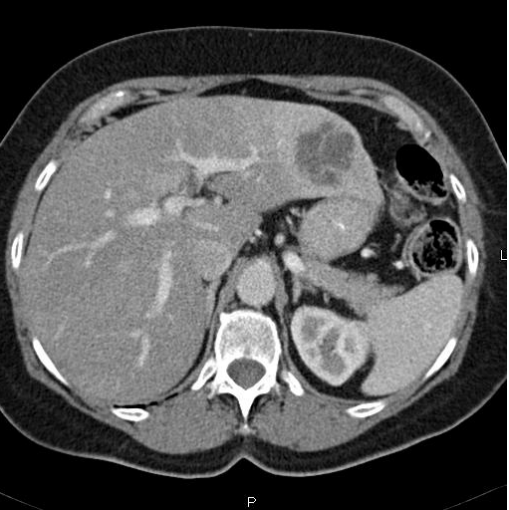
CT scan of the abdomen and pelvis; CT scan of the abdomen and pelvis with IV contrast demonstrates a 4.9 × 4.9 cm enhancing, poorly marginated mass in segment II of the liver.
